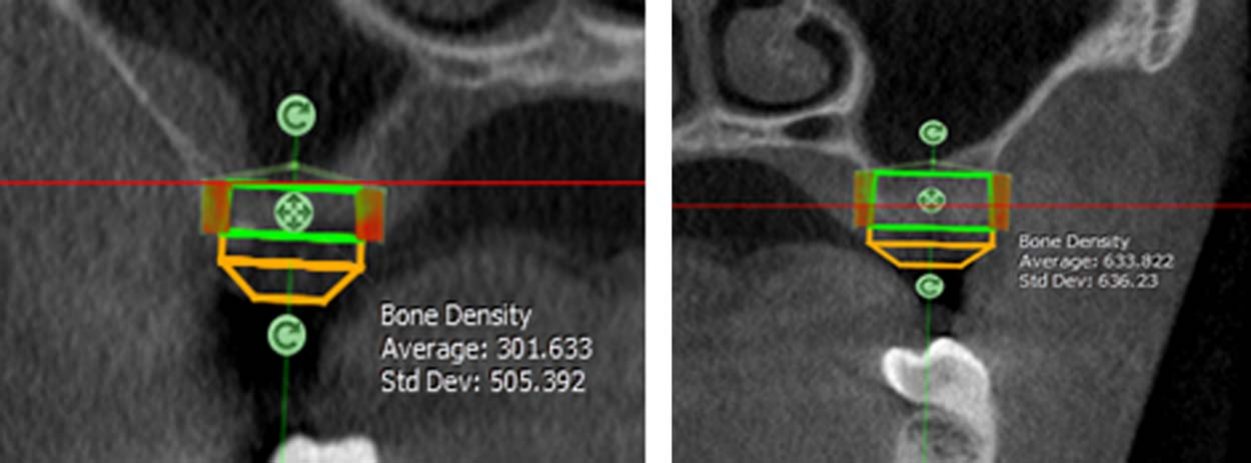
Shows measurement of bone density using CBCT scan (somatropin was applied in the socket of upper left molar.
